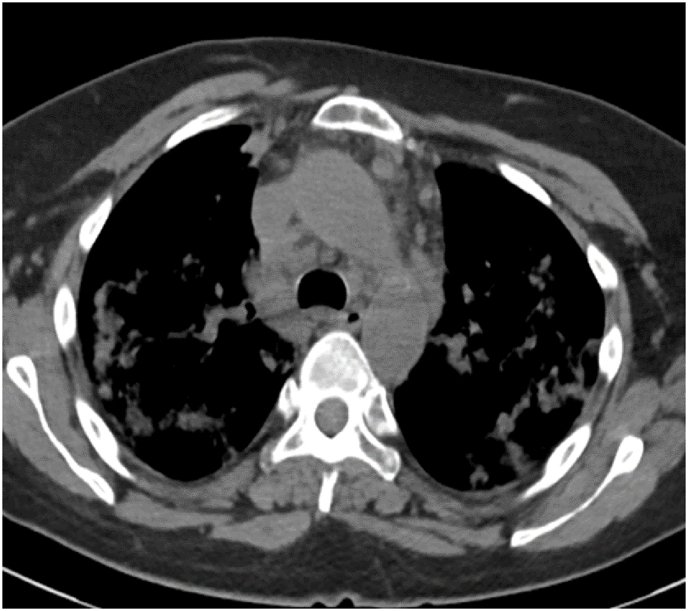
CT chest (mediastinal window), January 2019: there is multiple mediastinal enlarged lymph nodes.
